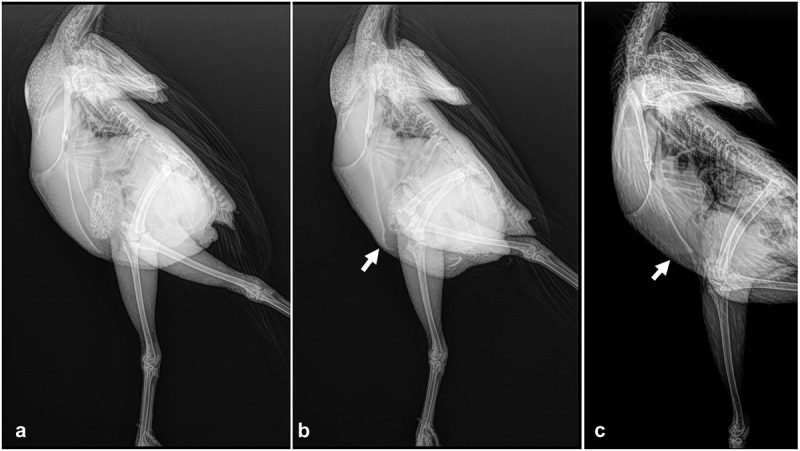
Three x-rays of living hens showing (a) a hen during the laying period with a normal keel, (b) a hen during the laying period with a distorted keel bone at the caudal tip of the keel which is indicated with an arrow, and (c) a pre-pubertal hen at 14 weeks of age showing the limited extent of the keel bone mineralisation with an arrow showing the caudal most extent of mineralisation.
